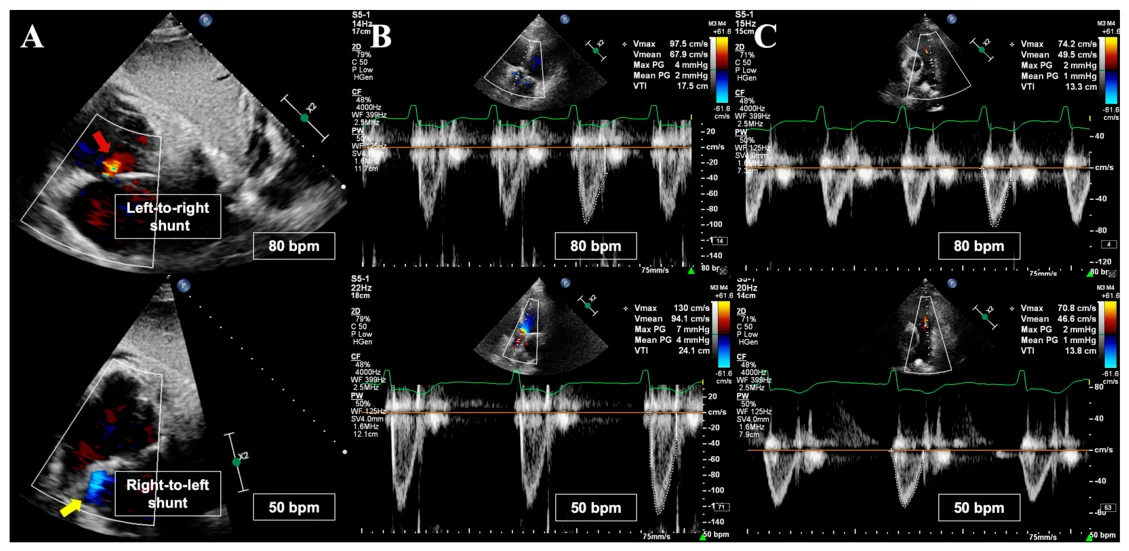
Heart rate-dependent interatrial shunting and biventricular hemodynamic mismatch. (A) Transthoracic echocardiography demonstrating dynamic shunting through a PFO: left-to-right at 80 bpm (upper panel, red arrow) and right-to-left at 50 bpm (lower panel, yellow arrow). (B) Pulsed-wave Doppler of the left ventricular outflow tract (LVOT, diameter 20 mm) showing VTI of 17.5 cm at 80 bpm (upper) and 24.1 cm at 50 bpm (lower); calculated stroke volume 55.0 mL and 75.7 mL, cardiac output 4.4 L/min and 3.8 L/min, respectively. (C) Pulsed-wave Doppler of the right ventricular outflow tract (RVOT, diameter 23 mm) showing VTI of 13.3 cm at 80 bpm (upper) and 13.8 cm at 50 bpm (lower); calculated stroke volume 55.2 mL and 57.3 mL, cardiac output 4.4 L/min and 2.9 L/min, respectively.
